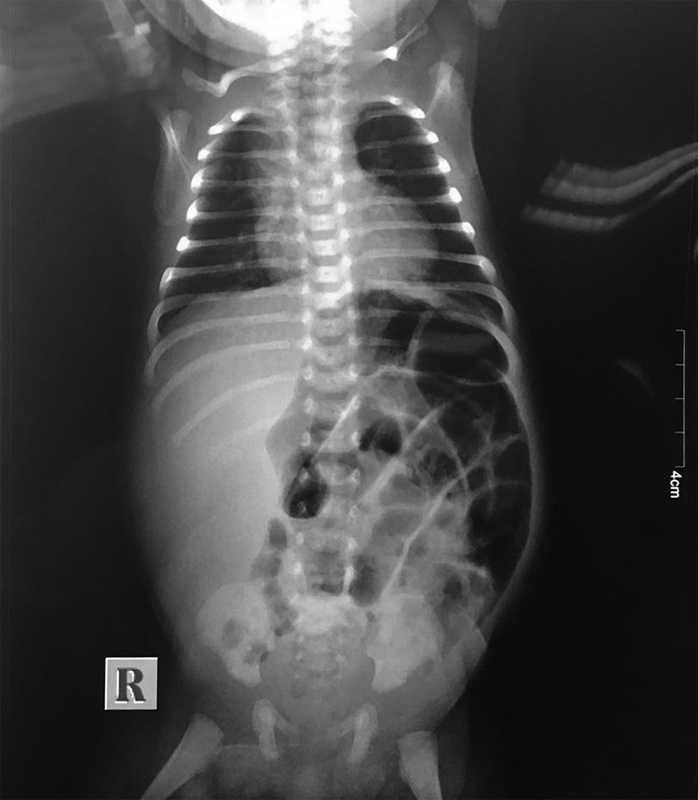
Preoperative abdominal X-ray.
